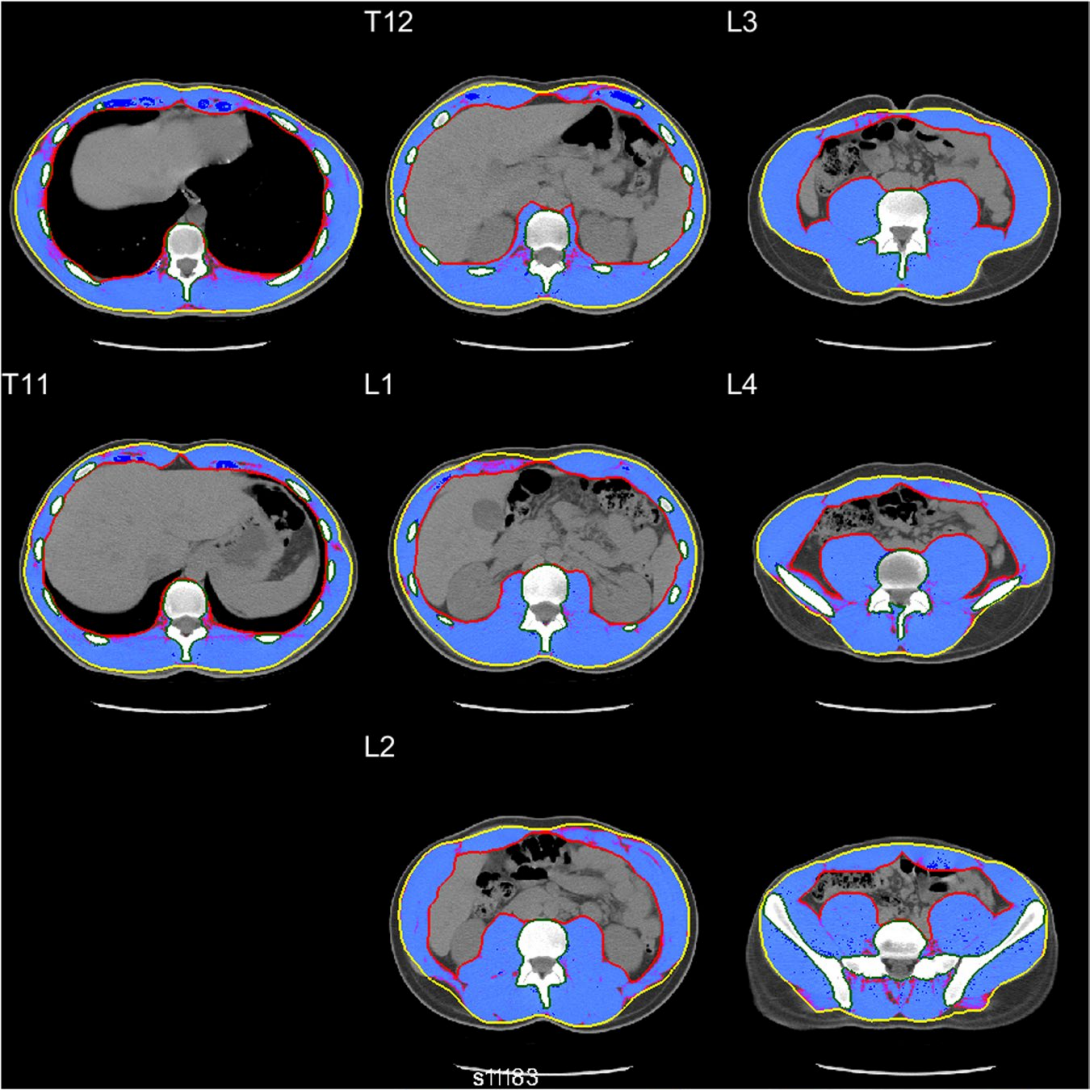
Example of healthy 20 y/o male T10-L5 axial CT slices showing SMA (blue-shaded area) between outer abdominal fascia (yellow line) and inner muscle wall (red line).
